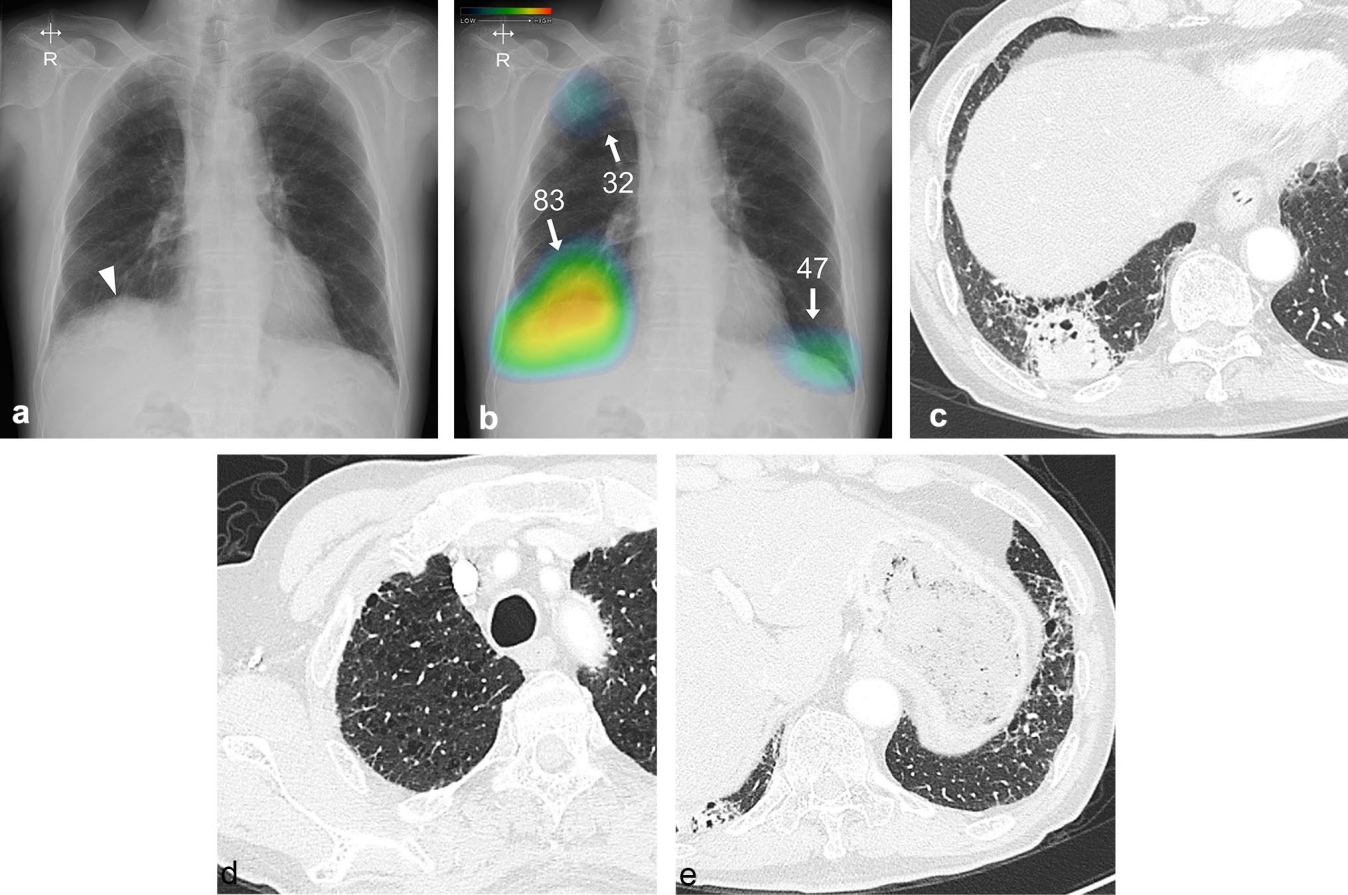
Diagnostic images of a 74-year-old male patient with squamous cell carcinoma in the right lower lung. a Chest radiograph showing a nodule in the right lower lung masked by the diaphragm (arrowhead), with a visibility score of 2 and a background lung score of 4. b The nodule was properly detected by the CAD with a probability of 83, with two non-target areas were detected with probabilities of 47 and 32, respectively. c Chest computed tomography (CT) showing a solid mass measuring 4.6 cm in size. d CT did not reveal any abnormality on the lesion with a score of 32, which was considered a non-target normal area. e CT revealed fibrosis on the lesion with a score of 47, which was considered a non-target abnormal area
